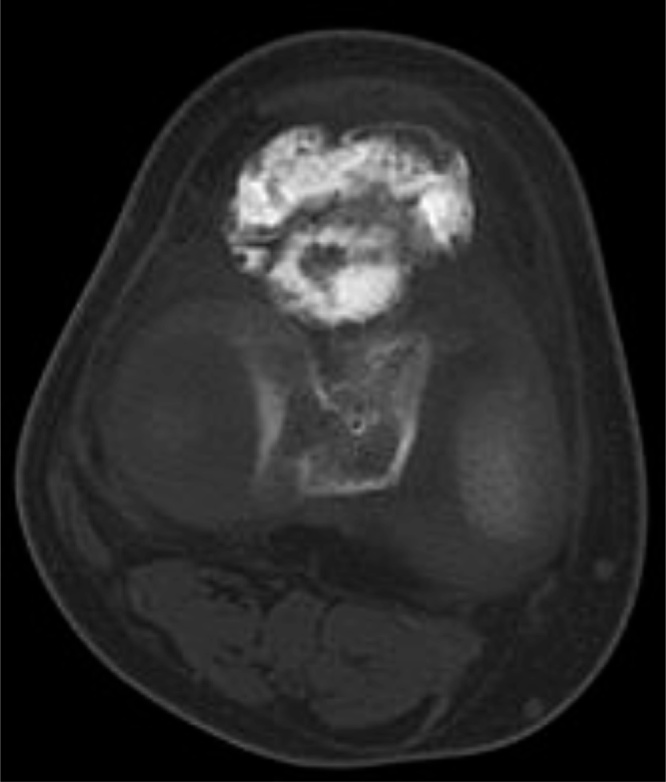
Computed tomography revealed an osseous lesion in the infrapatellar fat pad that was not attached to the bone or the articular cartilage.
